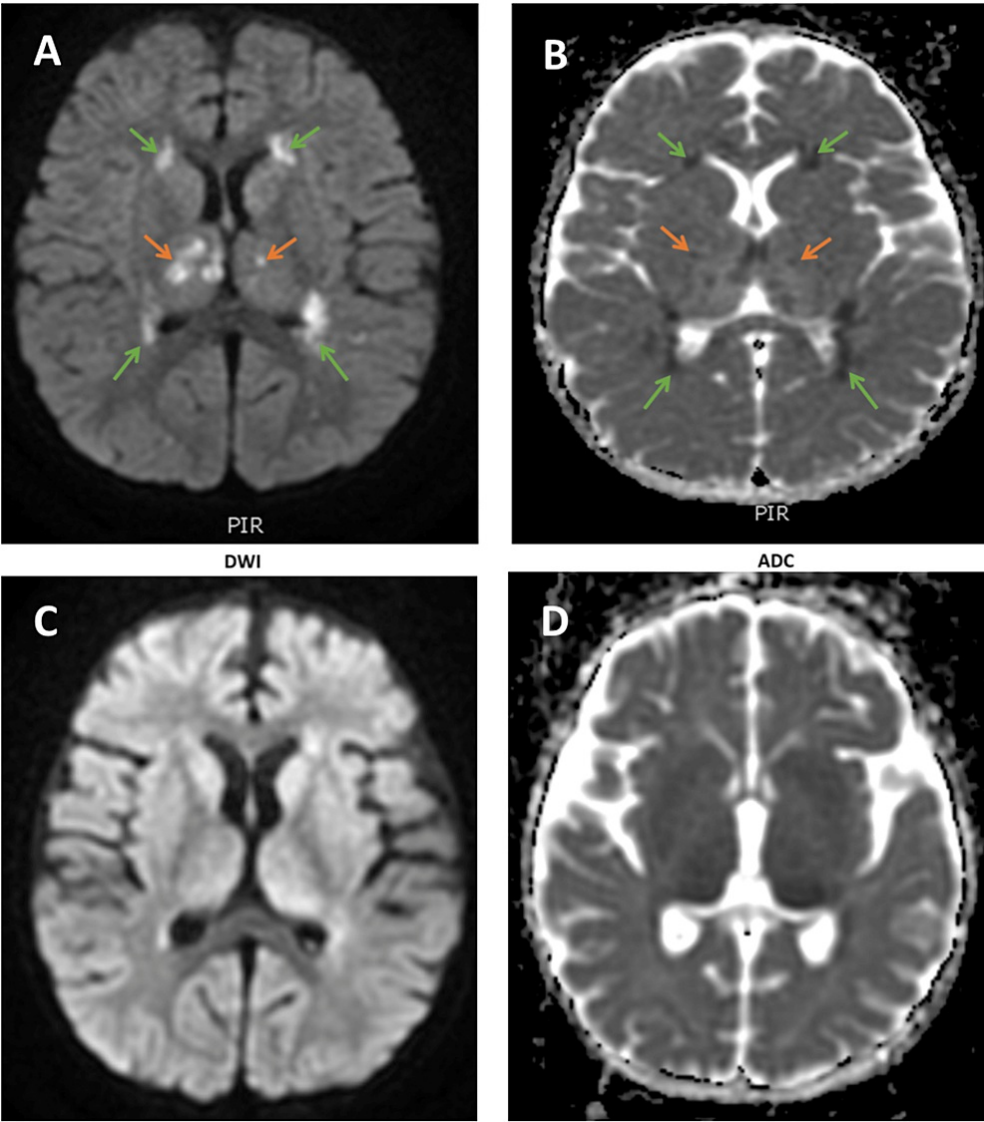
Initial brain MRI images Initial brain MRI images showed symmetrical bilateral periventricular (green arrows) and asymmetrical bilateral thalami (orange arrows) bright spots, which are visible on the axial image of the DWI modality (A). In the ADC modality (B), those same spots appear dark, which indicates limited diffusion and suggests cellular damage. In the repeated brain MRI images, the axial view of both the DWI modality (C) and the ADC modality (D) showed an interval resolution of restricted diffusion regions in bilateral deep periventricular white matter. DWI: diffusion-weighted imaging, ADC: apparent diffusion coefficient.
